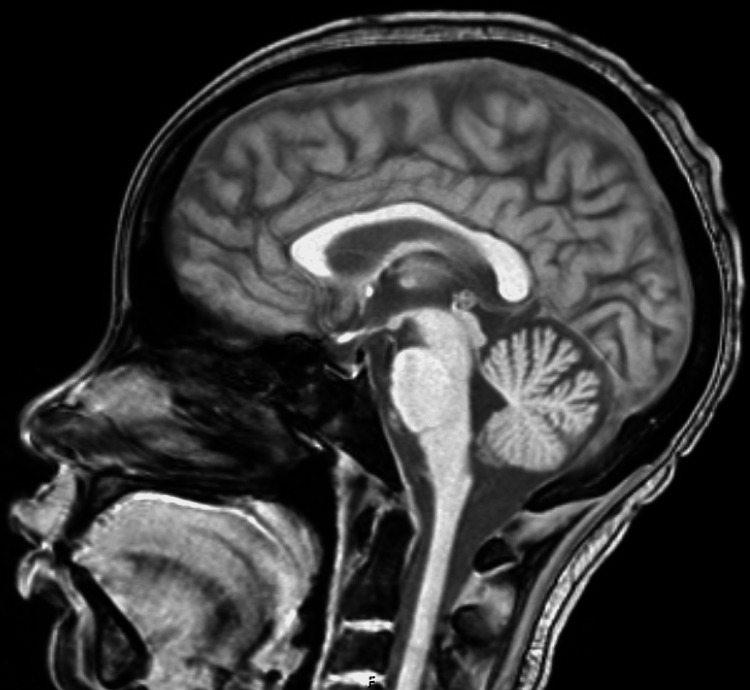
MRI of the brain with and without contrast with a T1-weighted image in the sagittal view showing a negative exam for acute infarct, intracranial hemorrhage, extra-axial collection, midline shift, or hydrocephalus. There is no abnormal enhancement or evidence of tumor. Major intracranial flow voids are present. There is normal enhancement of the major dural venous sinuses on high-resolution imaging. Overall, no acute intracranial abnormality.
